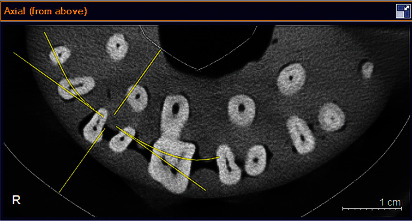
CBCT scan of maxillary molars.
